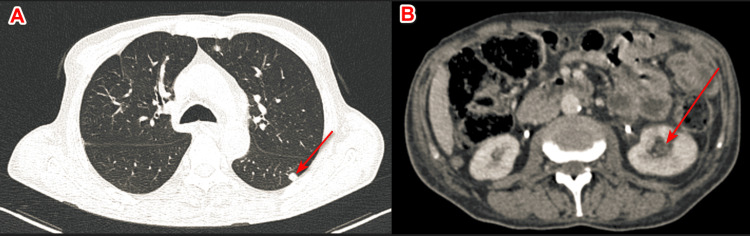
Axial section of abdominal and pelvic CT scan showing distant metastases of bladder tumor: sub-pleural and left lung parenchymal micronodule (A) and hypodense inferior polar left renal lesion with poor limitation (B) CT: computed tomography
